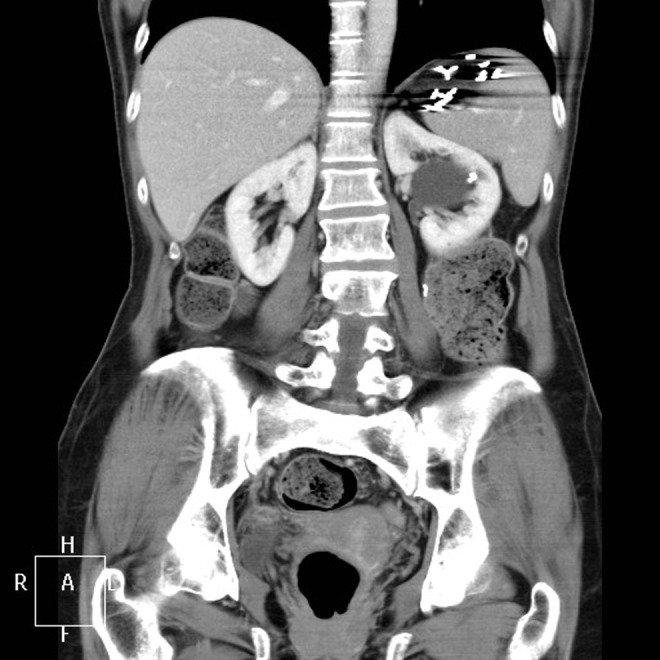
CT scan—coronal plane showing large renal cyst with renal calculus.
